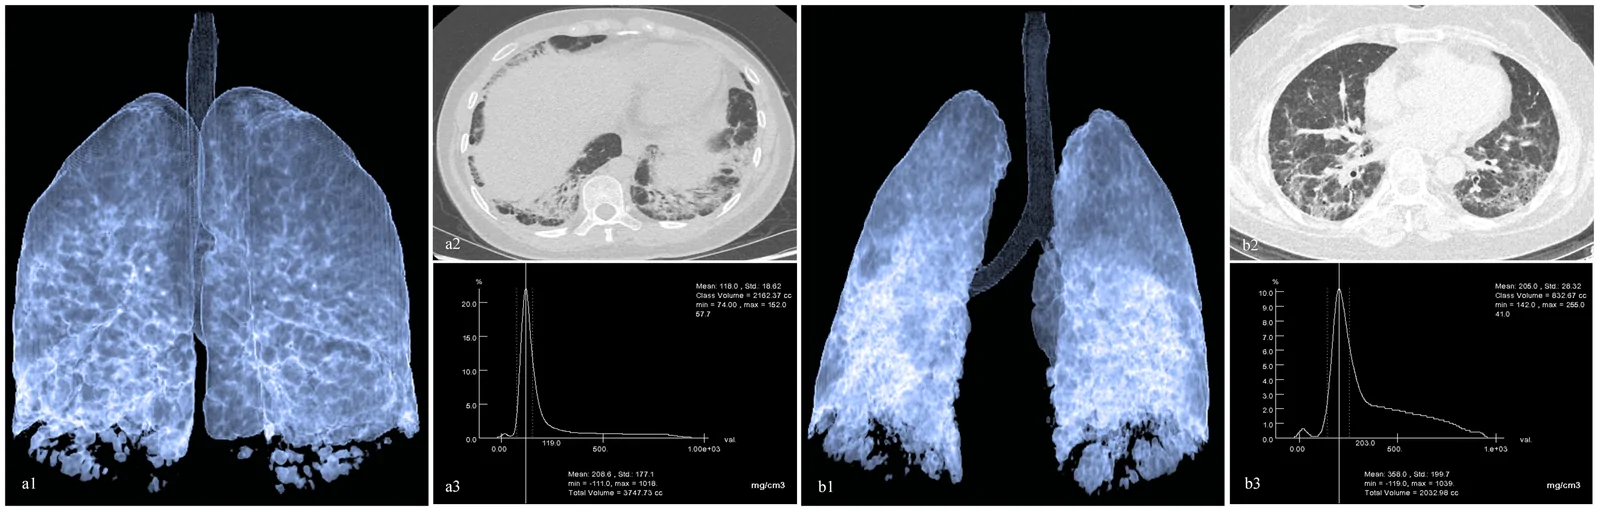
Representative imaging findings in NSIP. (a) Cellular NSIP (female, 49 years): HYP-ED 208.6 mg/cm^3^, lung volume 3747.73 cm^3^, FVC% Pred 82%, DLCO% Pred 80%. (b) Fibrotic NSIP (female, 53 years): HYP-ED 358.0 mg/cm^3^, lung volume 2032.98 cm^3^, FVC% Pred 70%, DLCO% Pred 65%. Panels: (1) VR image; (2) axial CT image; (3) HYP-ED distribution map.
